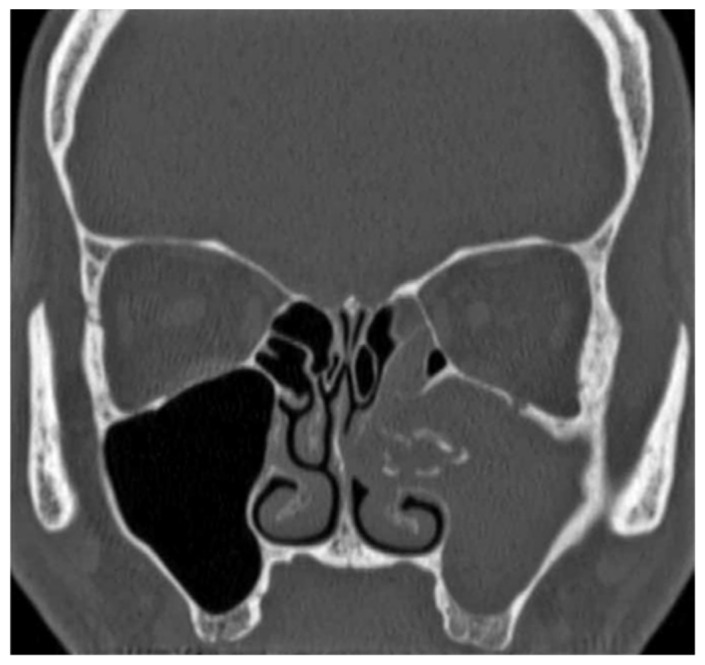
Coronal CT scan of the paranasal sinuses shows the left maxillary and ethmoidal sinuses were completely heterogonous opacified, with linear calcifications and hypertrophy of the maxillary sinus wall; fungal sinusitis.
